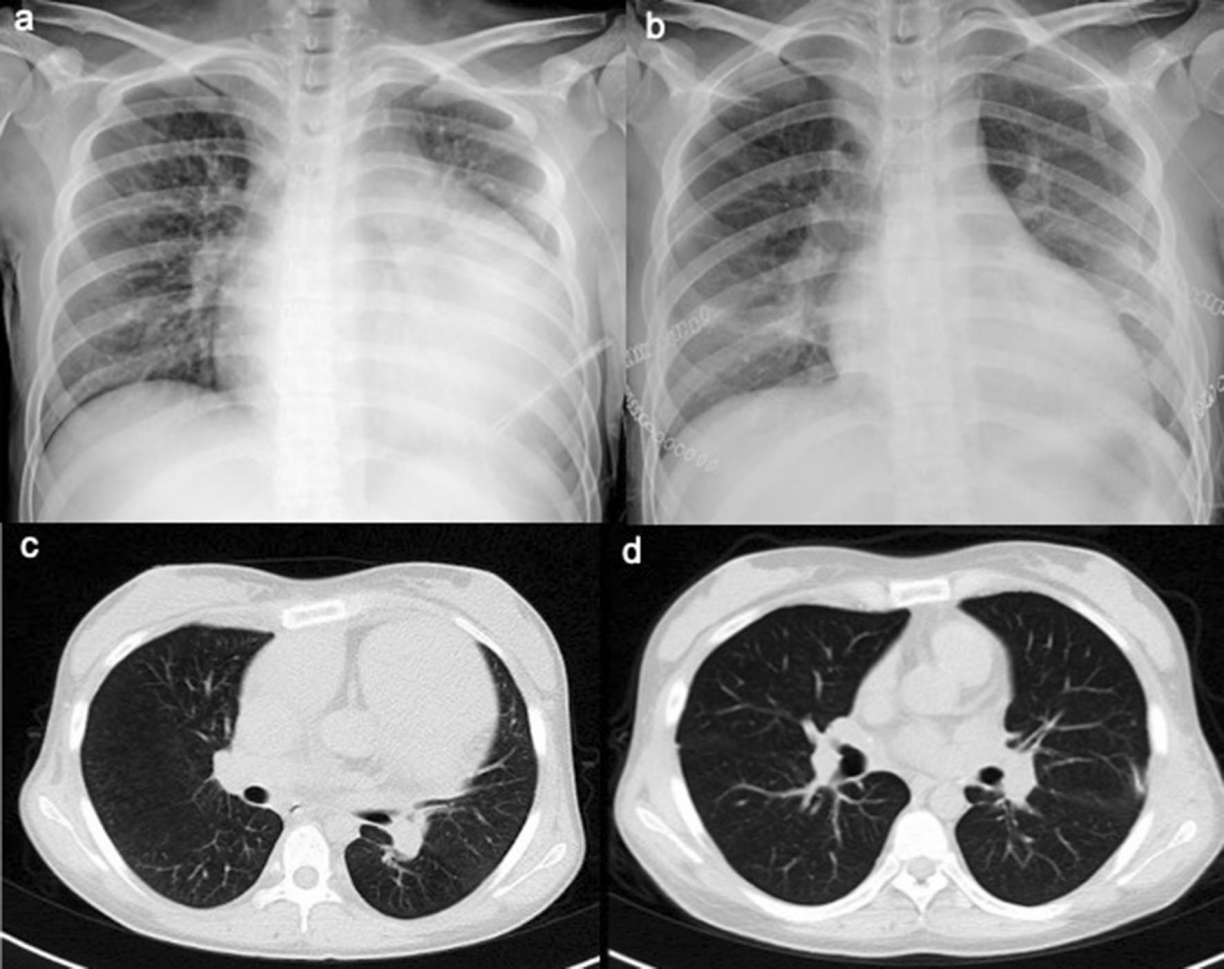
Radiogical examination. a, b: A chest radiograph of a child patient with IPAH was taken at 19 days before surgery (a). The chest radiographs of the IPAH child were performed one month after surgery (b). The CT examination of the IPAH child was implemented 5 months before surgery (c). The CT examination of the IPAH child was carried out 2 months after surgery (d). Comparisons of preoperative and postoperative images revealed significant improvement in IPAH after surgery.
